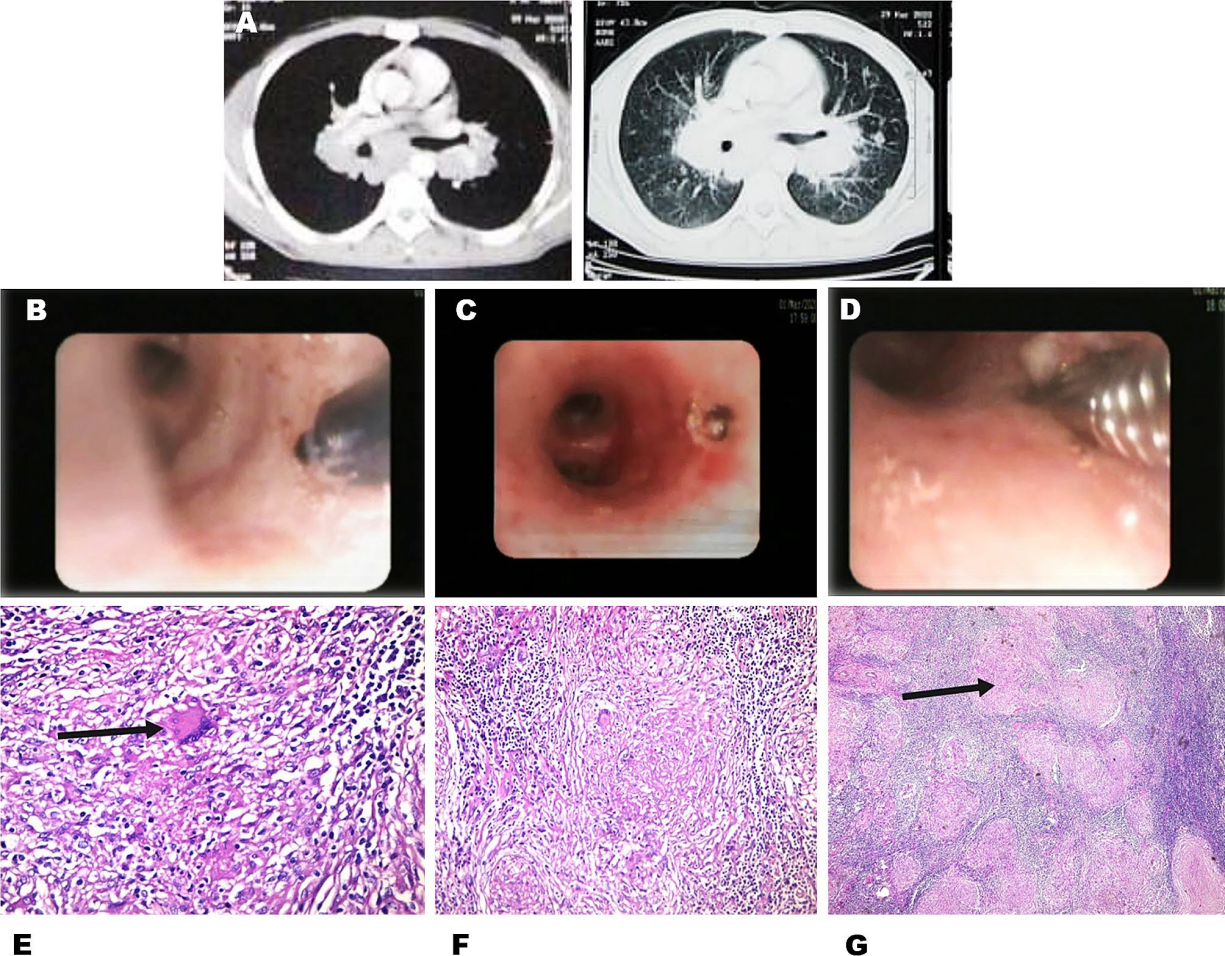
26 years old male, presenting with dry cough & dyspnea. CT chest with IV contrast (A) showing subcarinal and bilateral hilar discrete L.N with parenchymal nodules. The needle knife (B) was used to create the hole inside the bronchial wall (C). The forceps was advanced through the incision (D) and LN-TBFB was obtained from the enlarged subcarinal L.N. LN-TBFB image (high power) (E) showing multinucleated giant cells (black arrow) support the diagnosis of sarcoidosis. LN
